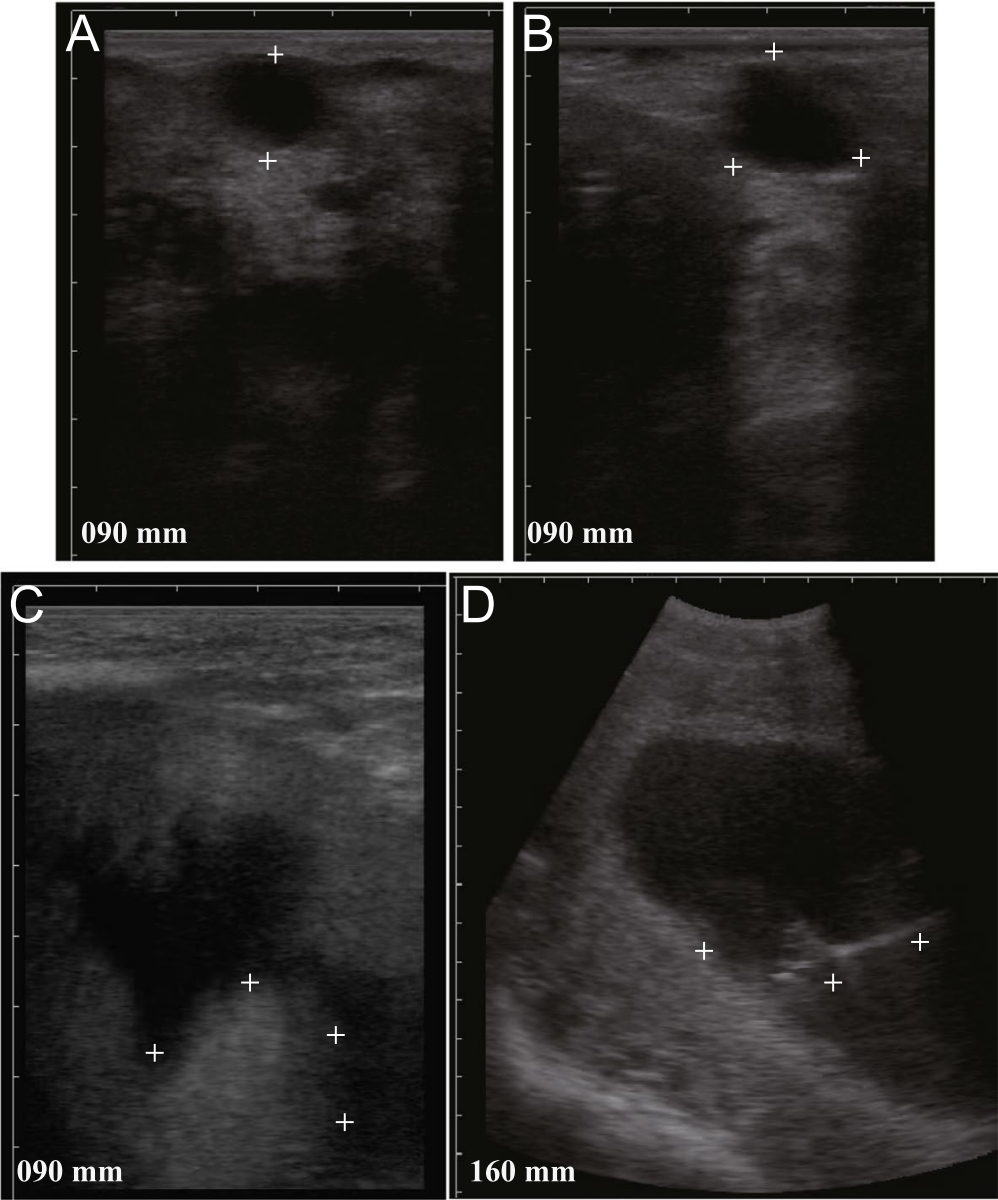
Ultrasound images of gestational sacs in Bactrian camel. A Transrectal ultrasonographic image of camel pregnancy 17 days after ovulation (6.5 MHz); B B-ultrasound imaging of camels at ~ 21 days of gestation (6.5 MHz); C B-ultrasound imaging of camels at ~ 90 days of gestation (6.5 MHz); D Transabdominal ultrasonographic images of camels at ~ 150 days of gestation (5.0 MHz)
